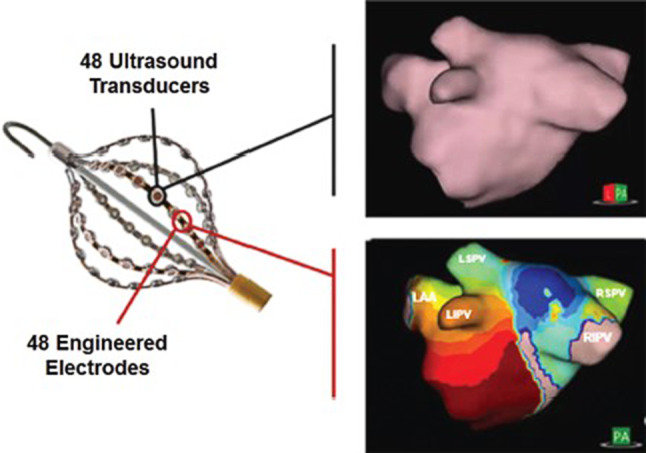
AcQMap catheter. The six splines of the catheter have 48 ultrasound transducers for anatomy reconstruction (upper right panel) and 48 electrodes for recording biopotential signals used to create propagation history maps (lower right panel). This figure was reprinted with permission from [10]
